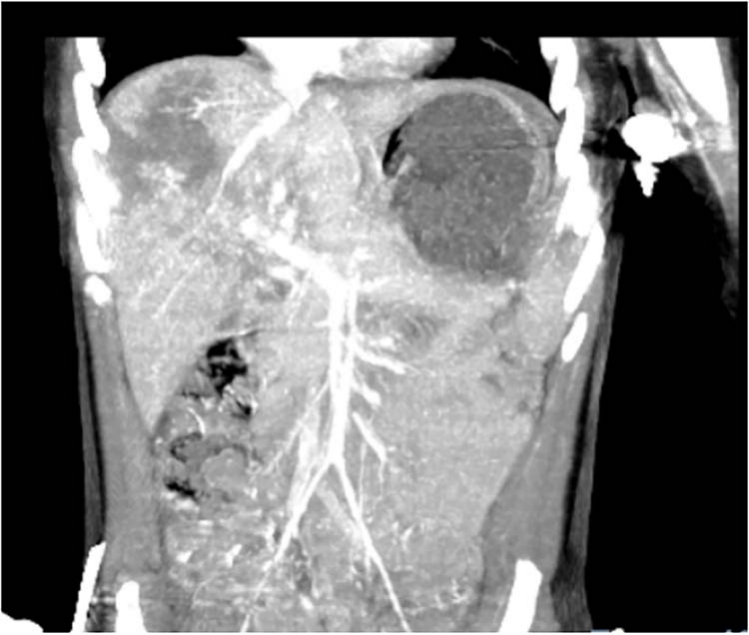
Coronal view of CT image revealing liver and splenic injuries.
